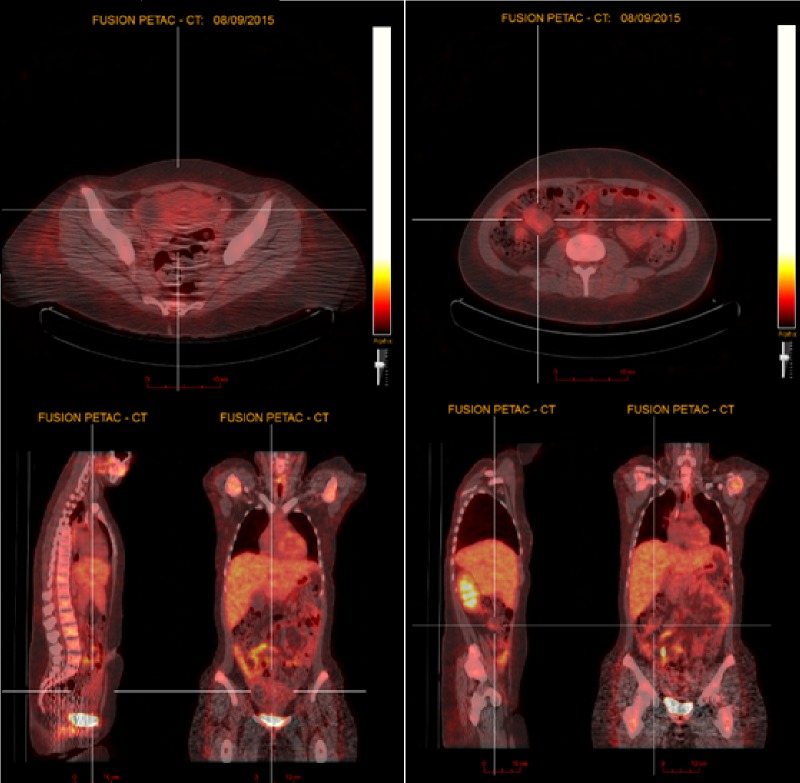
Re-staging PET-CT scan PET-scan after six cycles of chemotherapy showing excellent response with only two residual masses: one in the right flank with an SUV of 2.3, and a second in the pelvis with an SUV of < 2.
